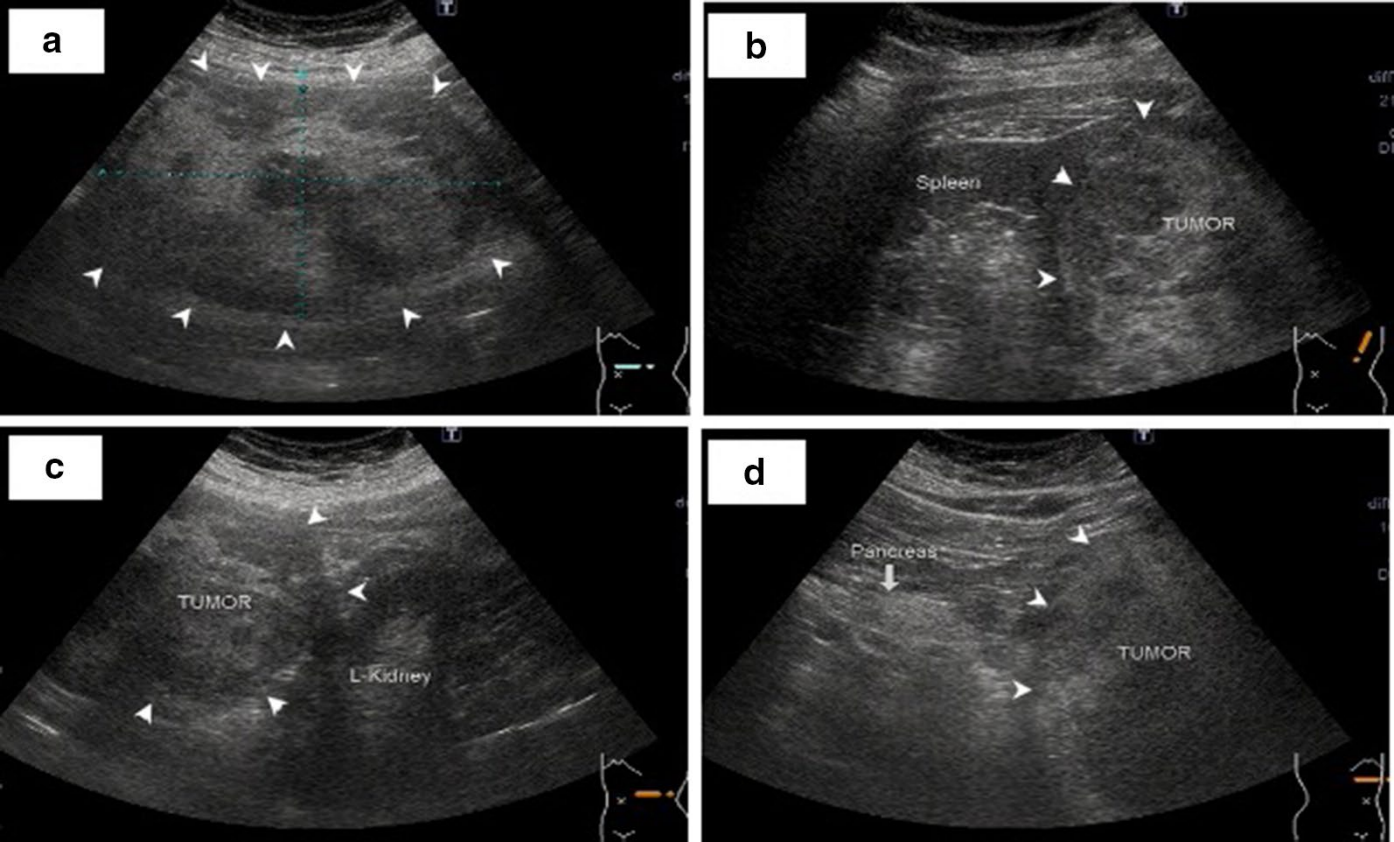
Abdominal ultrasonography. Arrows indicate the tumor. a A solid tumor measuring 140 × 128 × 99 cm in the upper left abdomen with uneven hyperechoic and hypoechoic areas. b The tumor was continuous with the upper pole of the spleen. c, d Although it was in contact with the left kidney and pancreas, it was discontinuous
